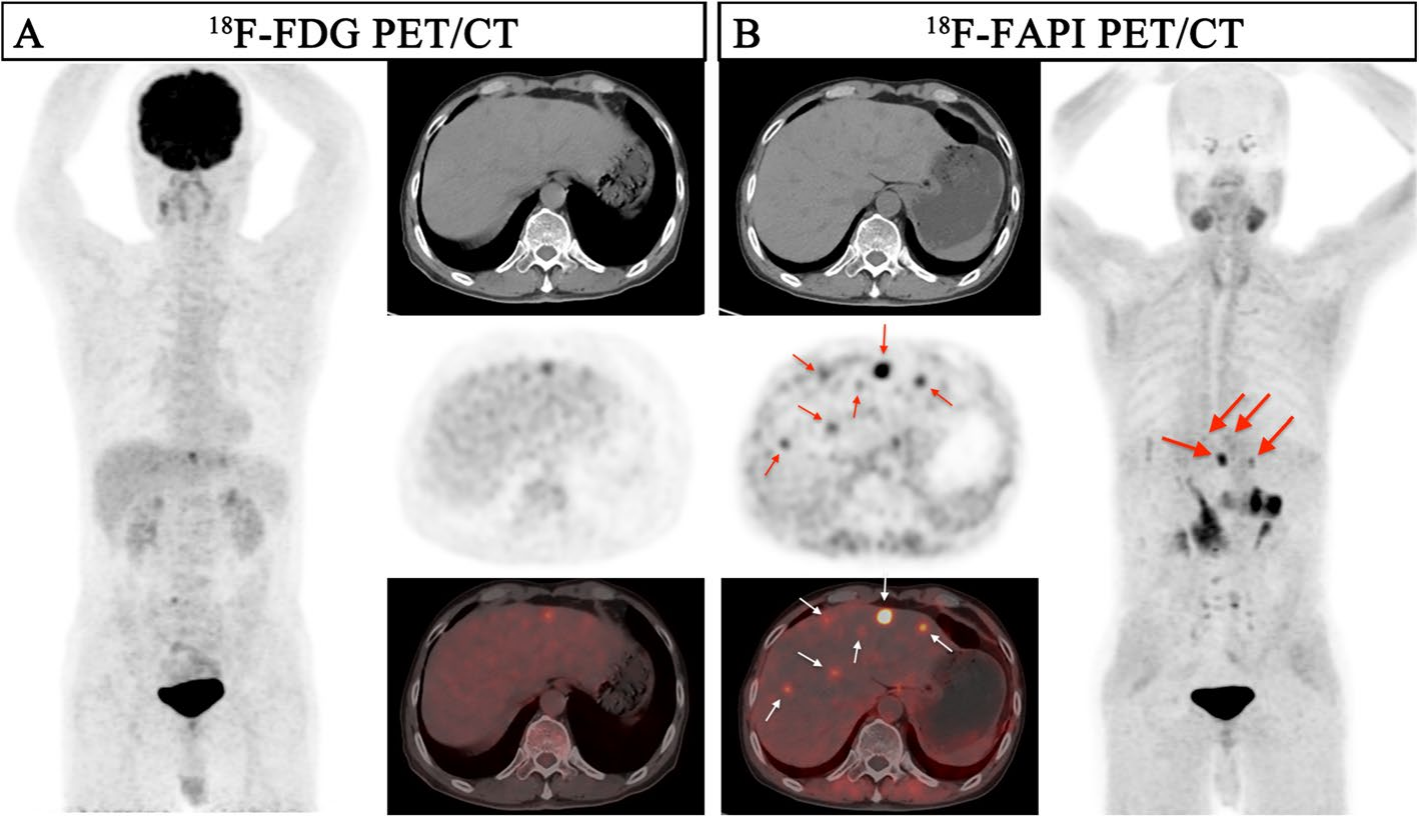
A 41-year-old male patient (Patient No. 51) with HCC (moderately differentiated) was confirmed by biopsy. ^18^F-FDG PET/CT displayed moderate uptake in the section II of the liver; However, the corresponding CT scan showed more nodules in other lobes of the liver. ^18^F-FAPI PET/CT detects greater radiotracer in primary lesions and other intrahepatic subfoci on both MIP (large arrow) and axial images (small arrow)
